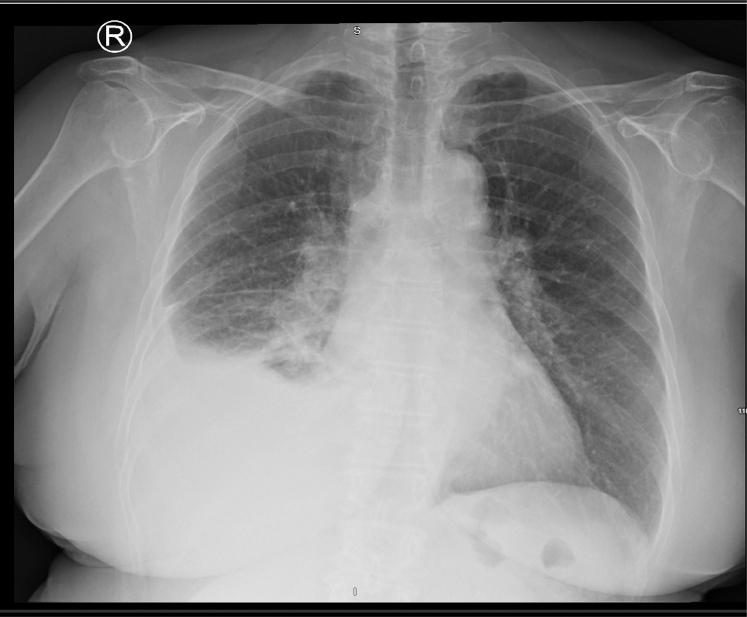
Posteroanterior(PA) chest radiograph showing moderate to large volume pleural effusion on the right side with some overlying consolidation.
